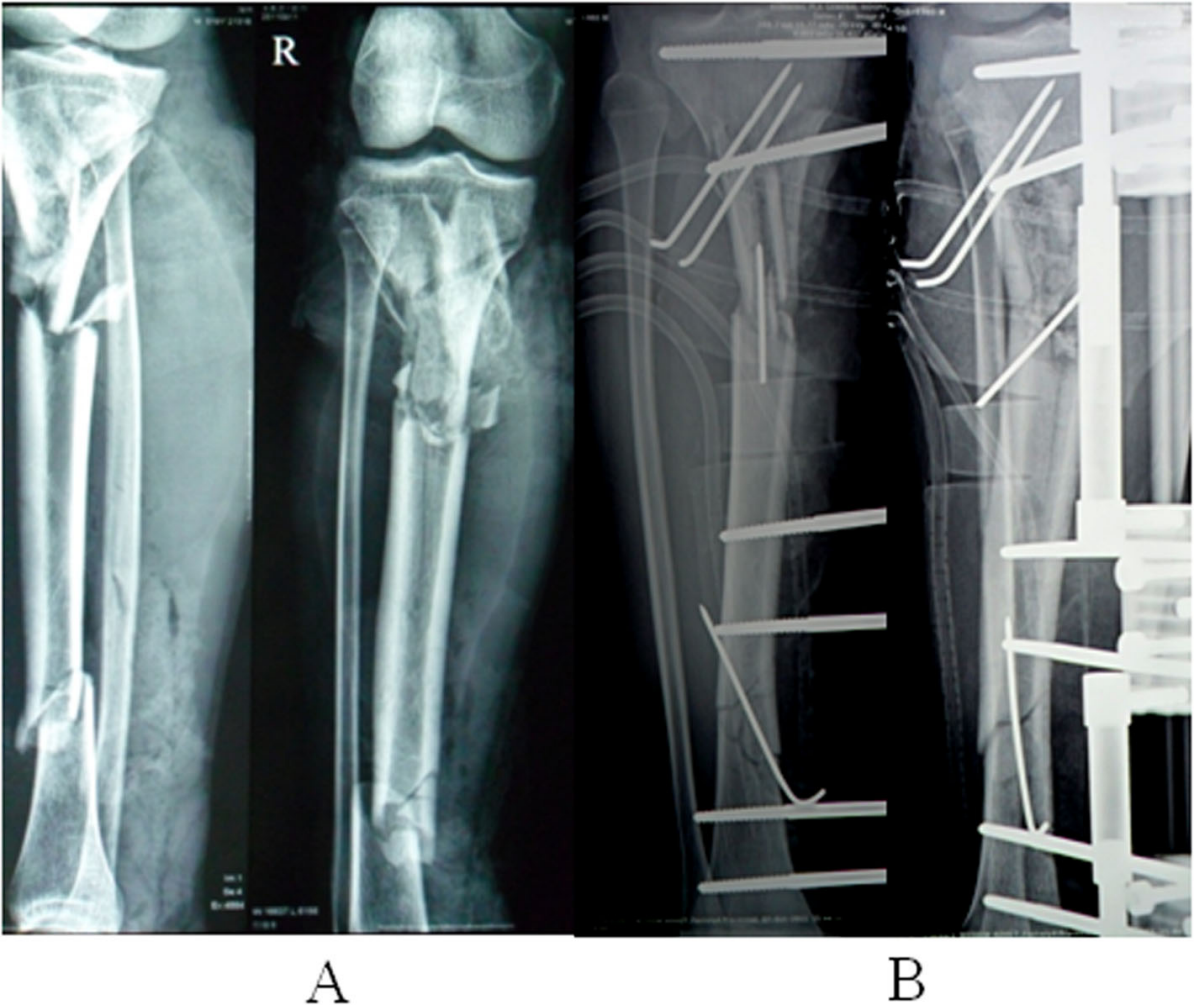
X-ray (a) at the time of injury and (b) following emergency debridement in the local hospital
